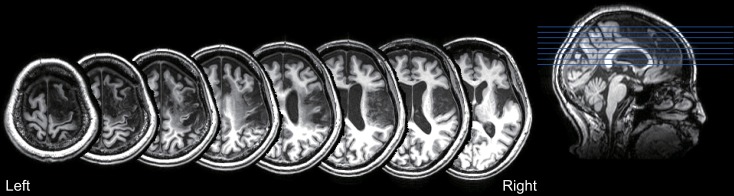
T1-weighted structural MRI of the patient. The lesion was observed in the fronto-parietal cortex, including the posterior parts of the inferior and superior frontal gyrus, anterior insula, anterior cingulate gyrus, pre and post central gyrus, and supramarginal gyrus. No lesion was observed in the left hemisphere.
